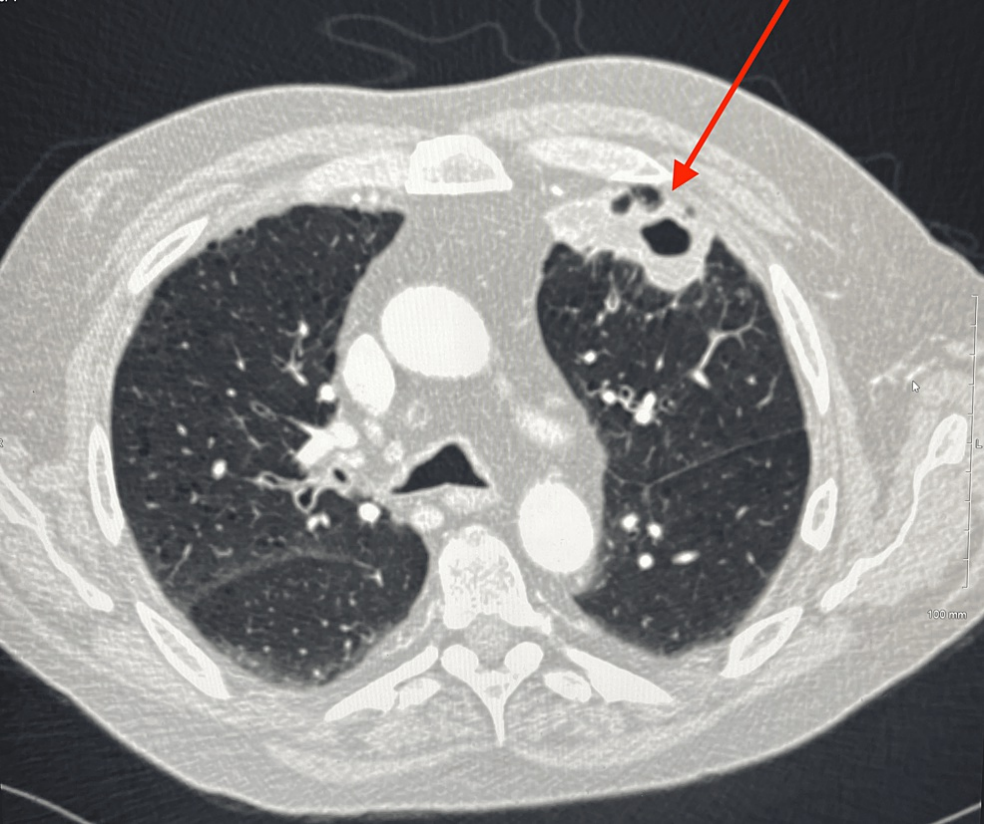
CTA of the chest showing a 6×3×6 cm partially cystic focal consolidation in the anterior left lobe. CTA: CT angiography
